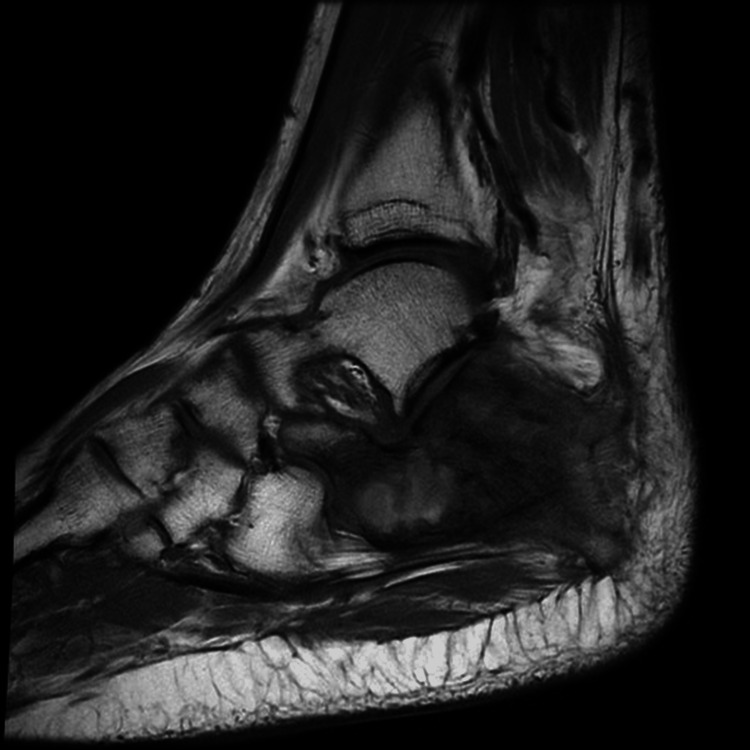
MRI of the left ankle (sagittal view) showing an extraosseous soft tissue mass surrounding the calcaneus.
